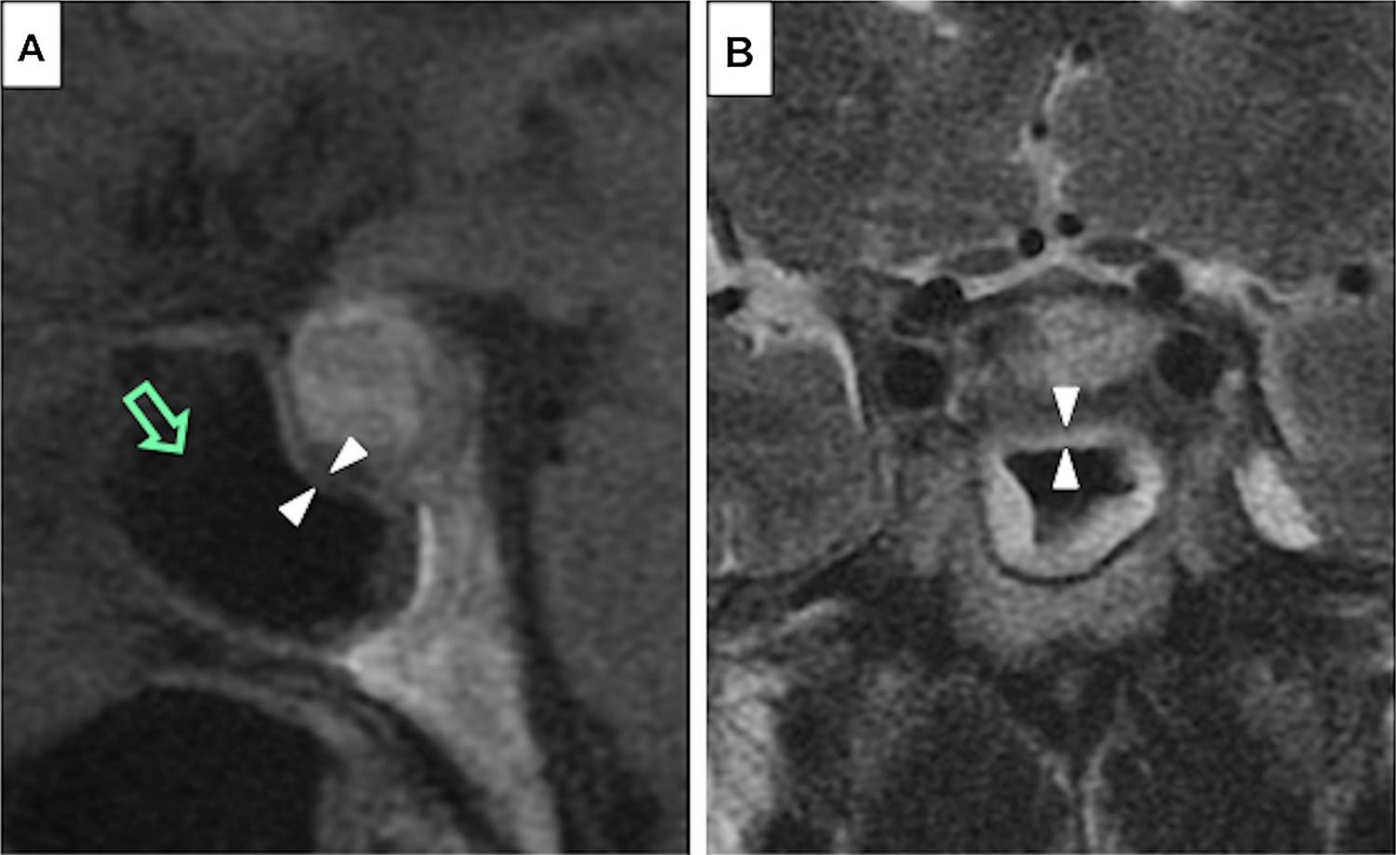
Sphenoid sinus mucosal thickness measurements were made as indicated by the white arrowheads on T1-weighted sagittal (a), or T2-weighted coronal MRI sequences (b). Sphenoid sinus opacification was assessed as the level of aeration of the sphenoid sinus (*green open arrow* in (a))
